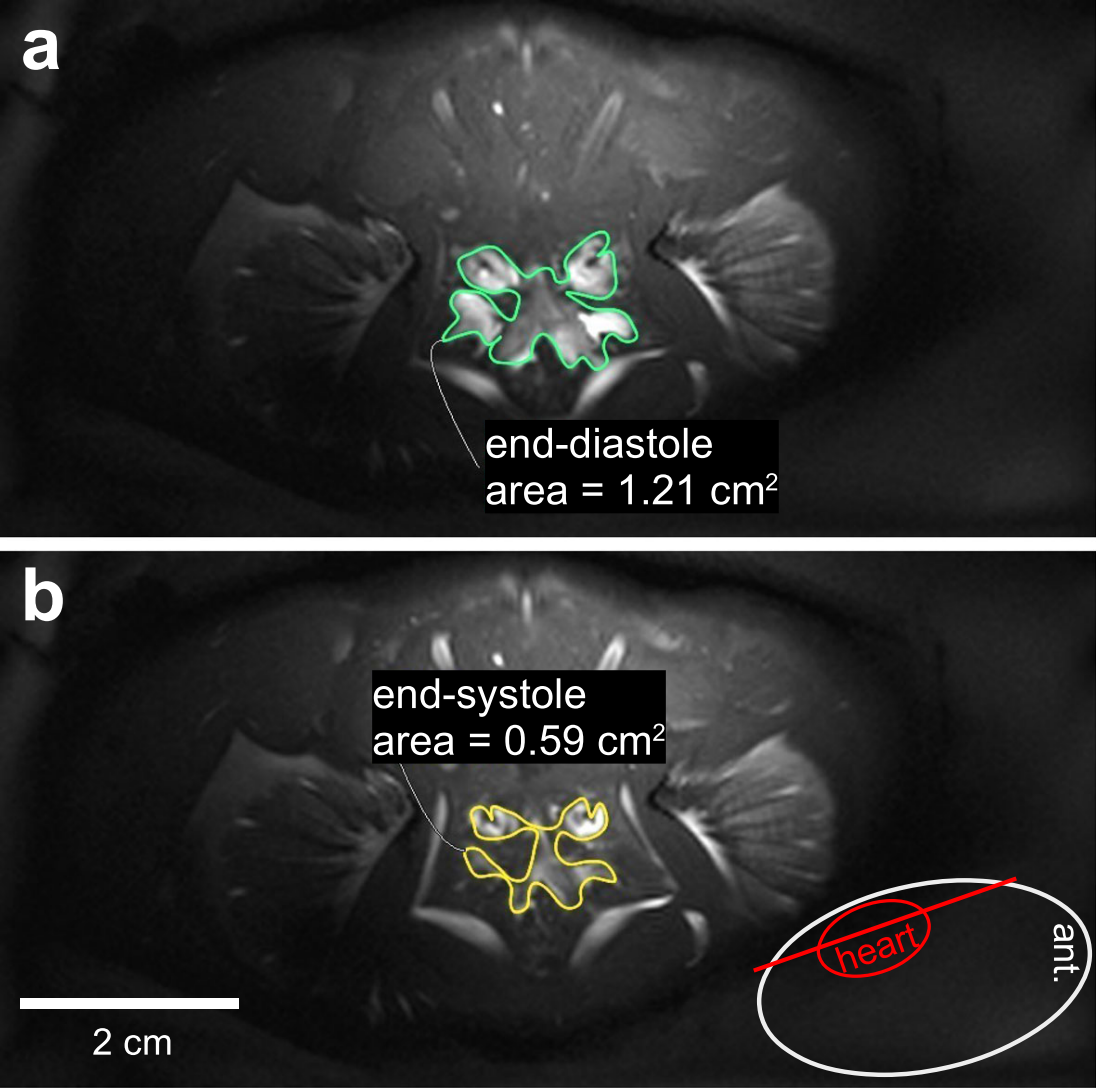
Examples of slice position for IntraGate© CINE MRI. a) End-diastolic and b) end-systolic frames from one IntraGate© CINE MRI scan. The lumen of the heart is outlined. The volumes are a) = 121 μL and b) = 59 μL. The slice position of the scan is depicted by the red line from a lateral view (anterior facing right)
